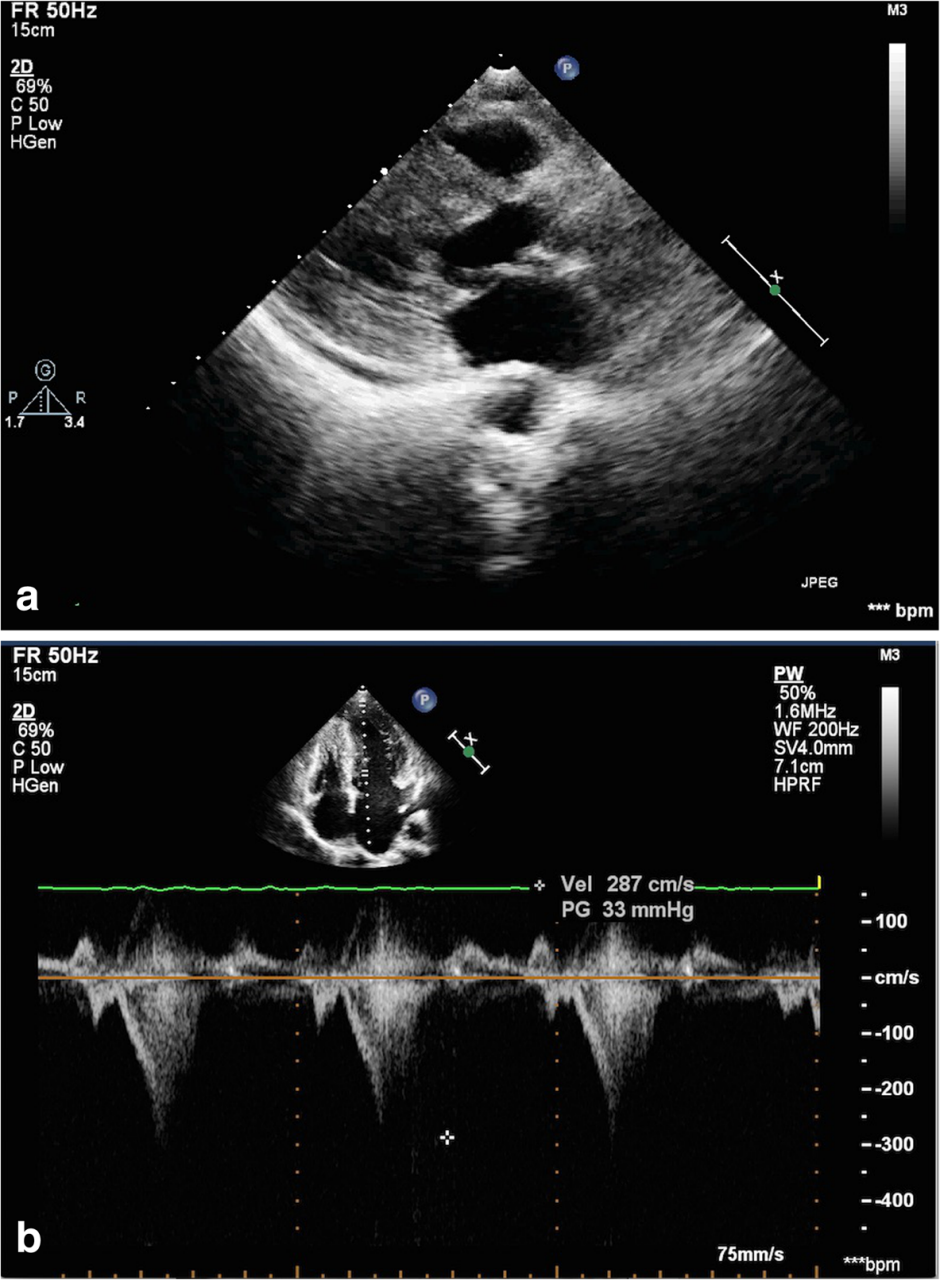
a Echocardiography revealed asymmetrical hypertrophic obstructive cardiomyopathy. b Pulsed wave Doppler suggested a resting sub-valvular obstruction with a pressure gradient of 33 mmHg
